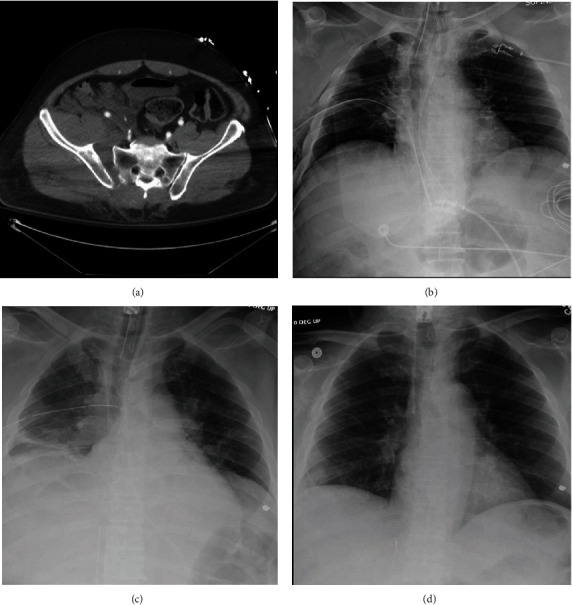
Patient 1 imaging including (a) admission pelvis CT, (b) admission chest X-ray, (c) chest X-ray while on ECMO, and (d) chest X-ray from the time of discharge.
